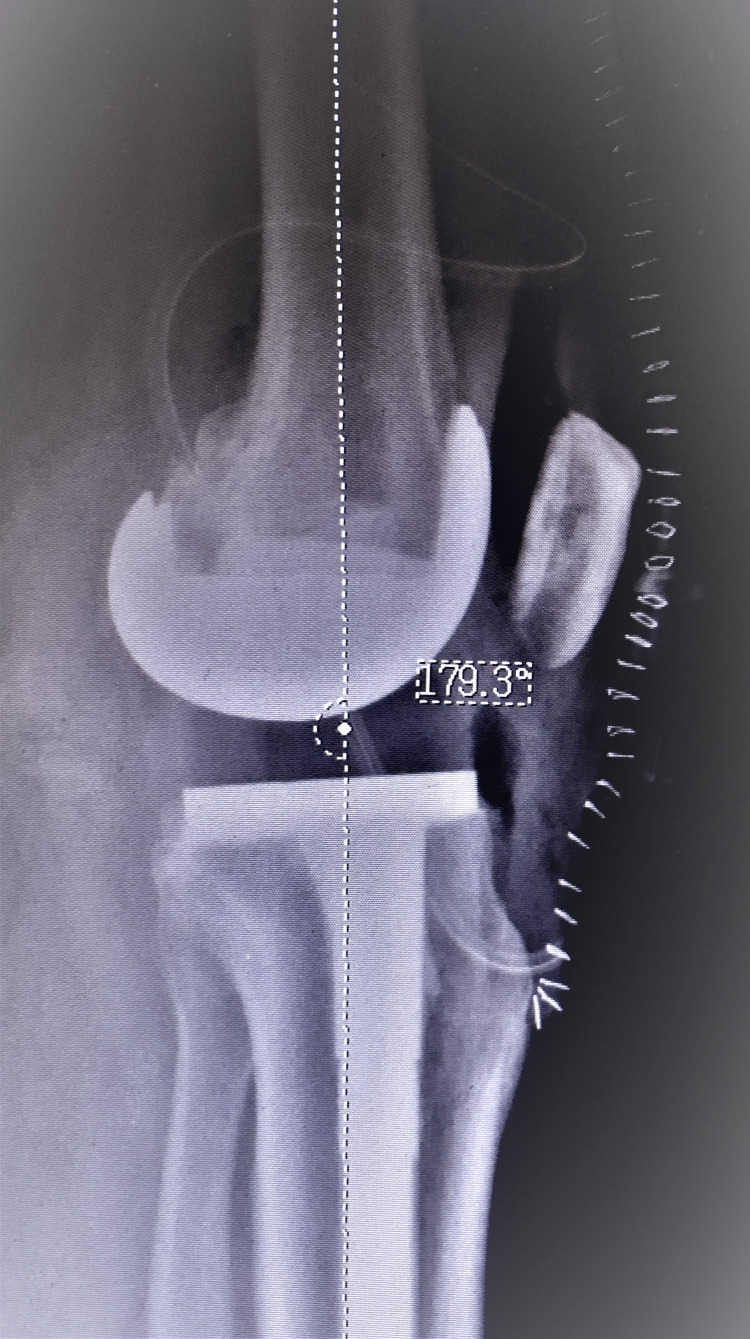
Immediate postoperative lateral view X-ray For the X-ray, consent and permission were obtained from the patient.
